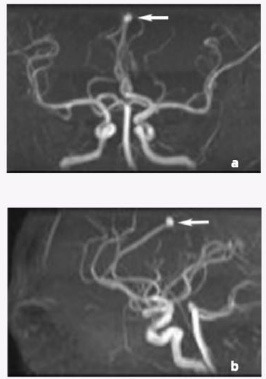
CT angiography of the patient on admission. (a, b) CT angiography shows an aneurysm (arrow) in the left cerebral artery at location A4.
